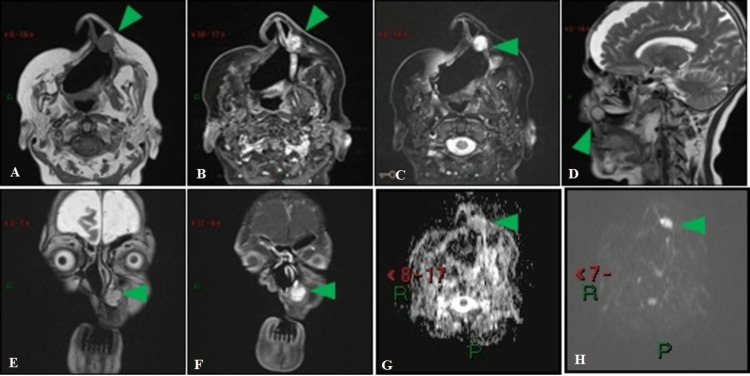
MRI seven years after the initial treatment. Contrast-enhanced MRI after extended maxillectomy, with reconstruction. Pre-contrast image (A) shows a lesion (arrowhead) with T1 hyposignal and heterogeneous contrast medium impregnation on post-contrast images (B, F). The lesion showed hyperintensity on T2 fat-suppressed and T2-weighted image (C-E) and low diffusion restriction (G-H). b-value in diffusion-weighted images = 1.000.
